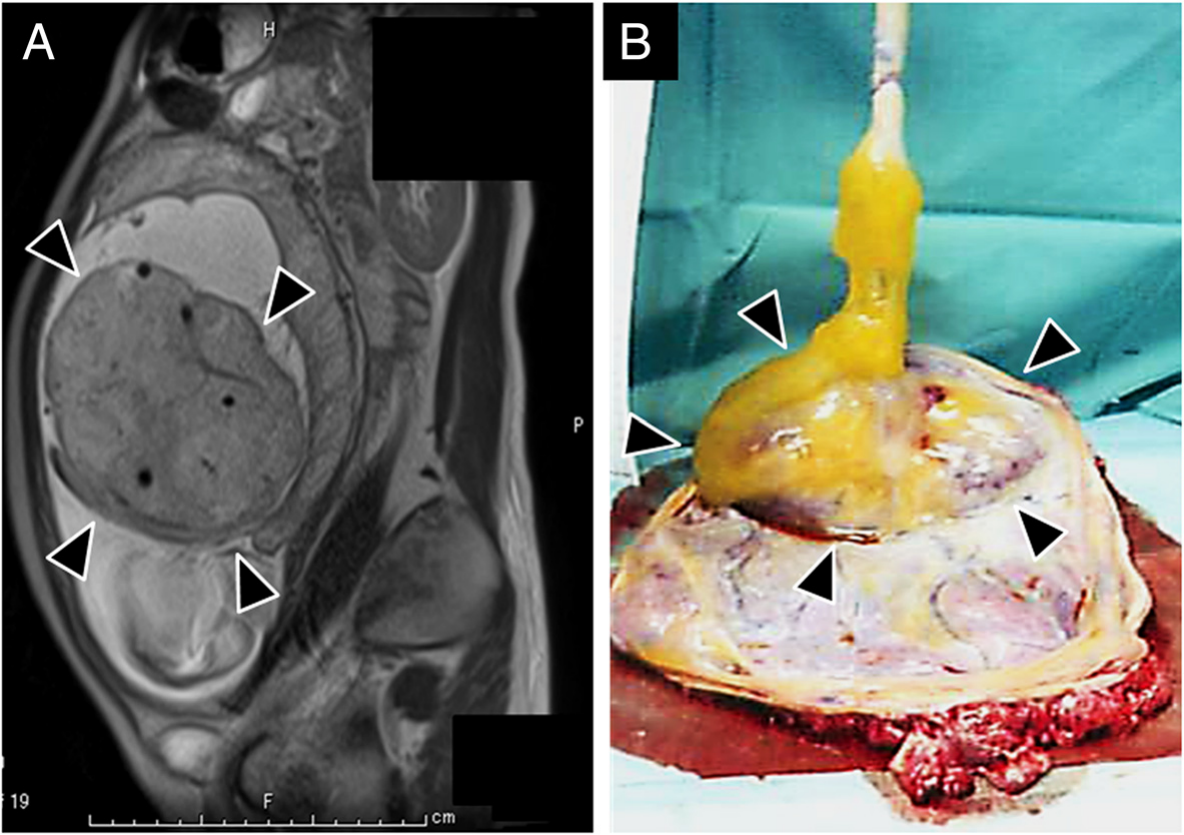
Clinical findings of cord hemangioma. a Fetal magnetic resonance imaging (MRI) performed at 27^th^ week of gestation. The solid mass lesion was identified close to but not attaching to the placental disc. Note the umbilical vessels are identified within the mass. b Macroscopic appearance of umbilical cord and placental disc before formalin fixation. The large and solid mass is seen at the placental end of the umbilical cord (Arrow heads)
